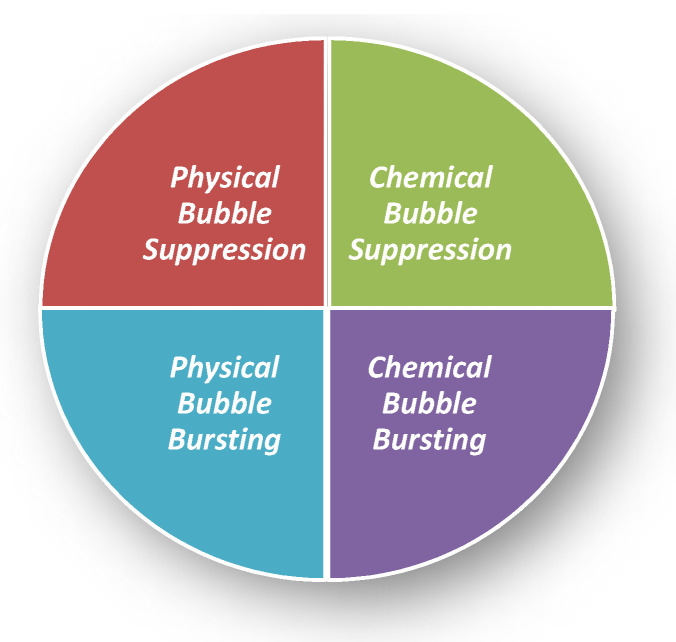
Four kinds of defoaming methods. Physical Bubble Suppression: Temperature change [22]; filtering to remove floating materials [21]; making the vessel open, to remove the mechanical foaming factors (to avoid violent boiling, oscillation, decompression, splashing). Physical Bubble Bursting: Temperature change [22] (freezing, heating [23], evaporation, drying); pressure change [24] (ultrasonic wave [25] and air injection); liquid injection; stirring and tapping with a hydrophobic metal mesh. Chemical Bubble Suppression: Adding defoaming agents; adding defoaming gases; using low foaming surfactants; adding electrolytes; adding substances that eliminate foam stability [21]. Chemical Bubble Bursting: Addition of electrolytes or by electrolysis to weaken the repulsion of the double electrical layers, adding substances discharging liquid [21]; salting out [26].
